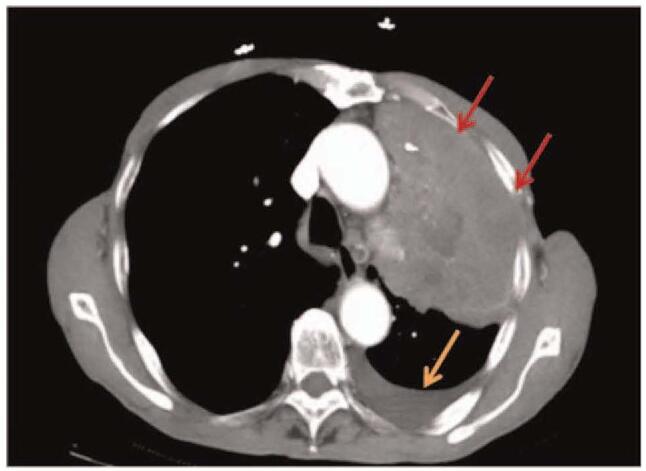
Computed tomography at the first presentation showing a 12.5-cm massive pulmonary tumor in the left upper lobe (Mediastinal window). The red arrows indicated lung masses and the yellow arrows indicated pleural effusion.
